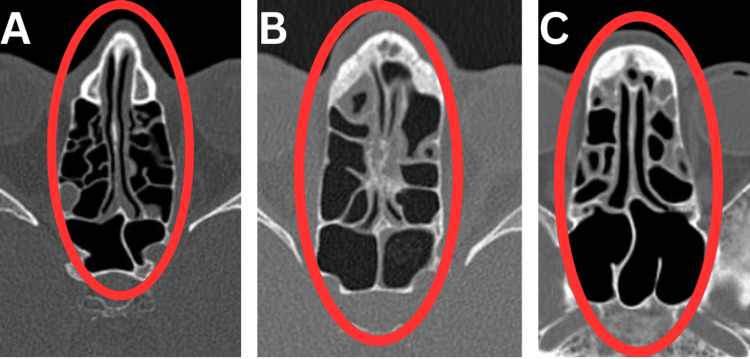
Axial non-contrast computed tomography (CT) images of the paranasal sinuses (PNS) highlighting the extent of sinus involvement. (A) Opacification of less than 25% of the ethmoid air cells is seen. (B) Opacification of 25-50% of the ethmoid air cells is seen. (C) Opacification of 50-75% of the ethmoid air cells is seen. Red ovals highlight the ethmoidal air cells, with the degree of sinus involvement estimated visually.
